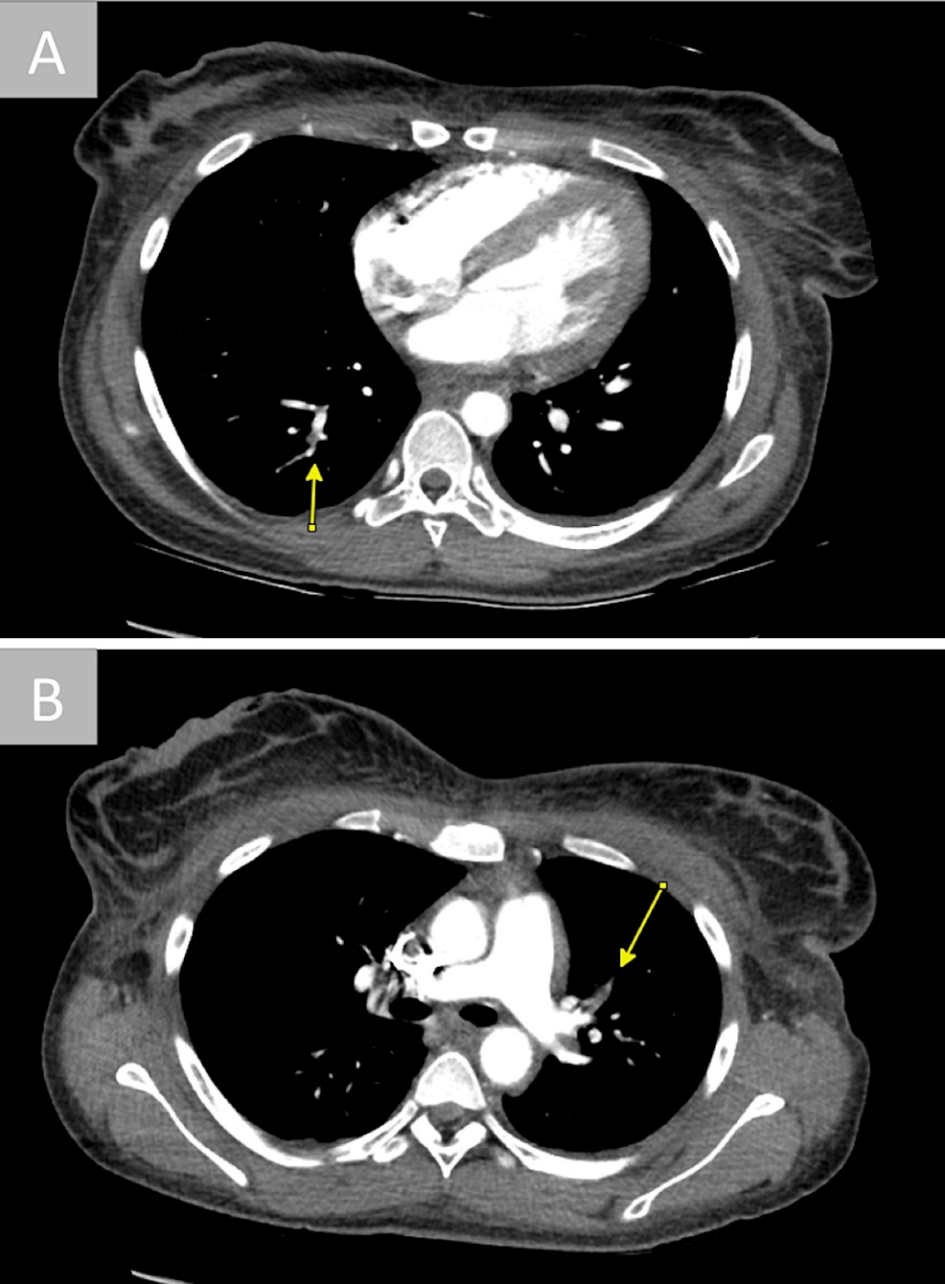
Computed tomography of the chest with pulmonary embolism protocol (CTA PE) The images show (A) right lower lobe posterior basilar segmental and subsegmental and (B) left upper lobe anterior segmental and subsegmental pulmonary emboli.
